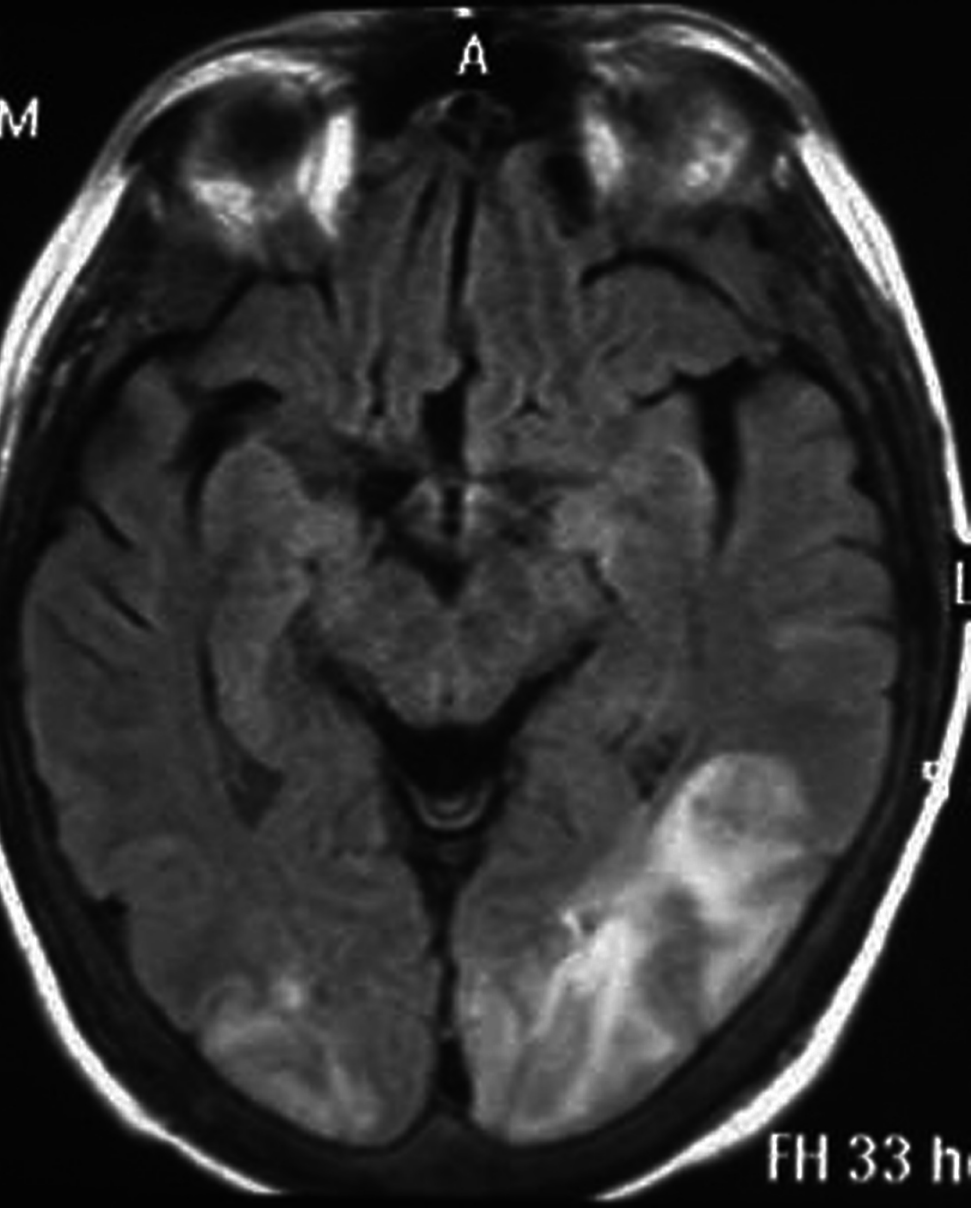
Brain MRI FLAIR Bilateral parieto-occipital hyperintese lesions.
